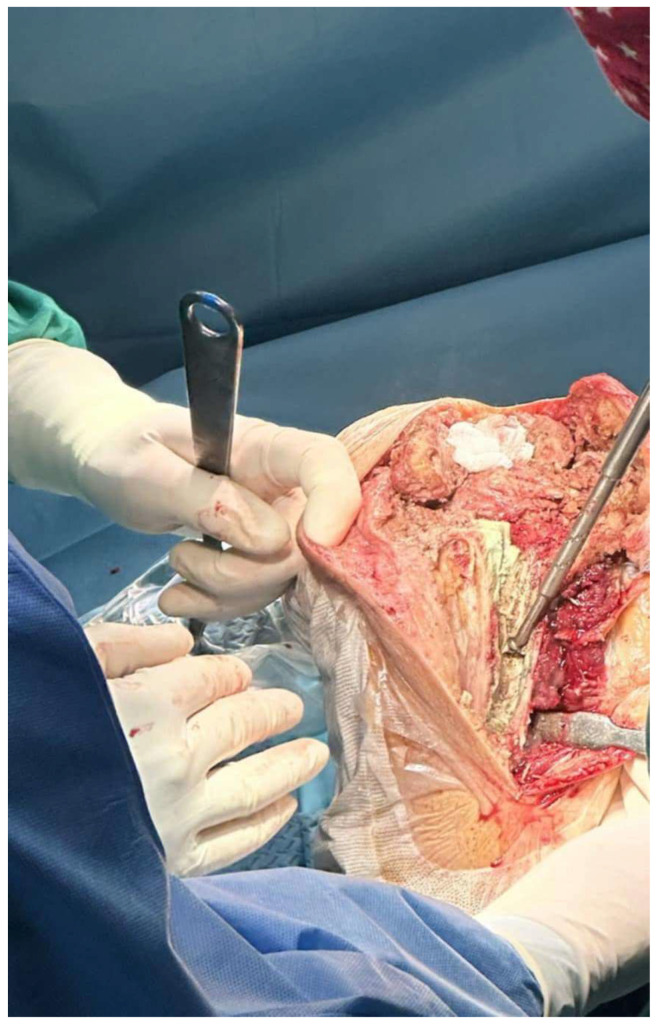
Removal of bone cement from intramedullary canal after the extraction of tibial stem with the use of an ultrasound probe (Case 3).
